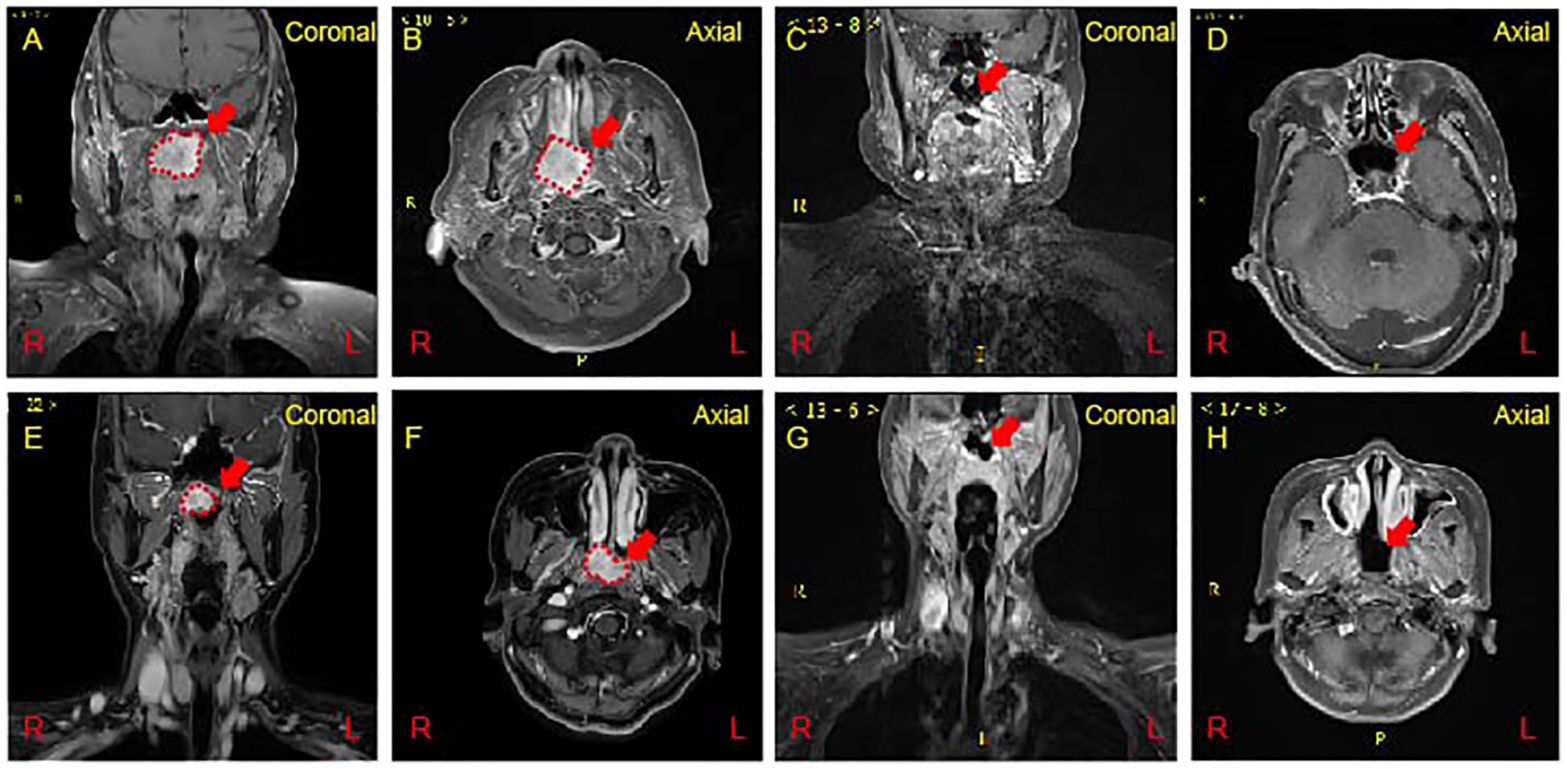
Magnetic Resonance Imaging (MRI) of the nasopharynx. (A, B) A polgs of Case 1 on MRI. A mass measuring approximately 38 * 32 mm was observed on the right side of the nasopharynx (Red dash line). Enhanced scanning reveals significant and uneven enhancement. (C, D) At 2.5 years post-treatment reveals the absence of any abnormal signal changes in the nasopharynx (Red arrow show). (E, F) An irregular mass measuring approximately 24 x 20 x 19 mm on the right side of the nasopharynx (Red dash line). Enhanced scan indicating significant and uneven enhancement of the nasopharyngeal mass. (G, H) Follow-up at 8 months post-treatment showing no abnormal signal changes in the nasopharynx (Red arrow show).
